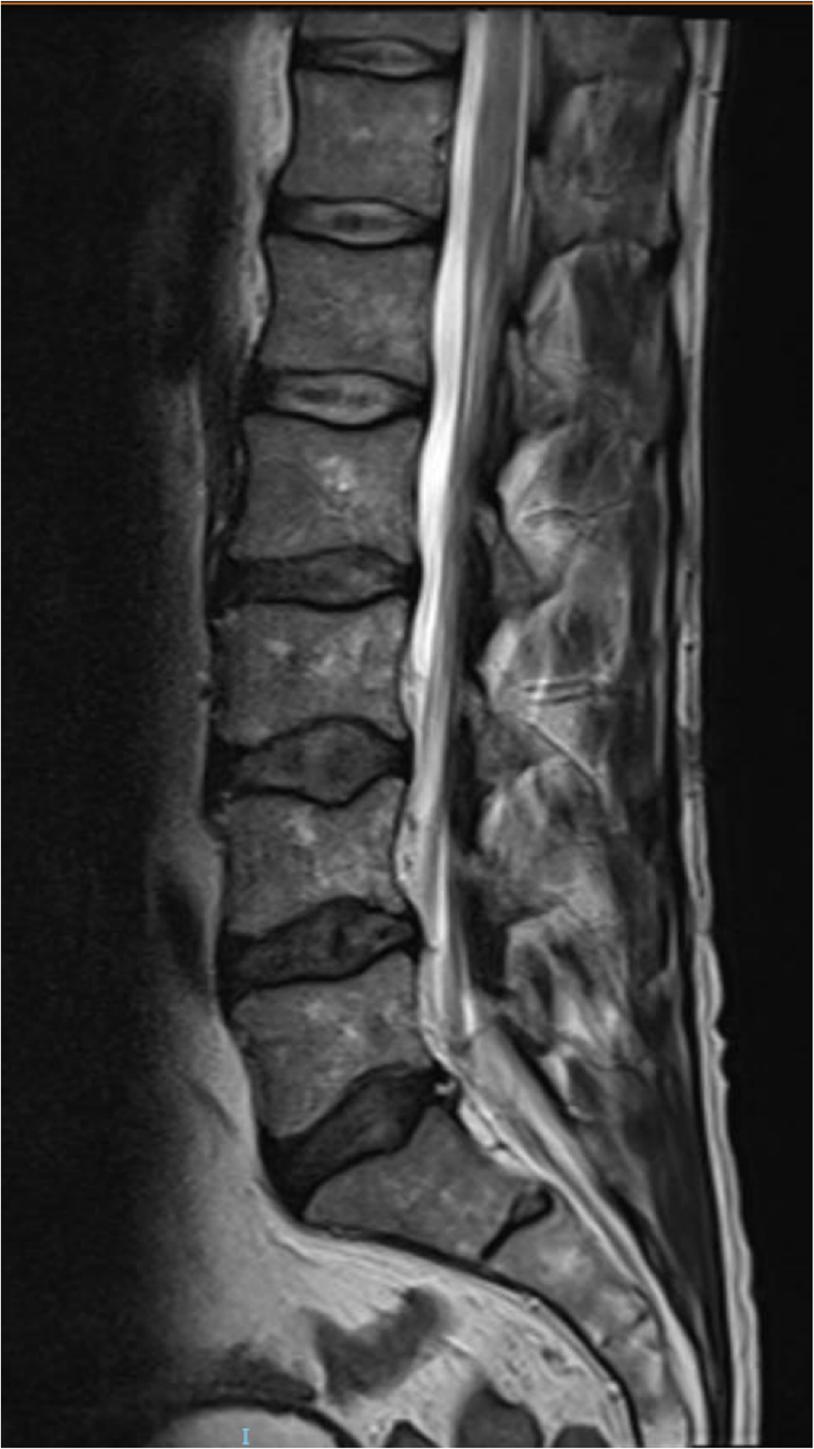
Magnetic resonance imaging of the lumbo-sacral spine showing no significant spinal cord compression
